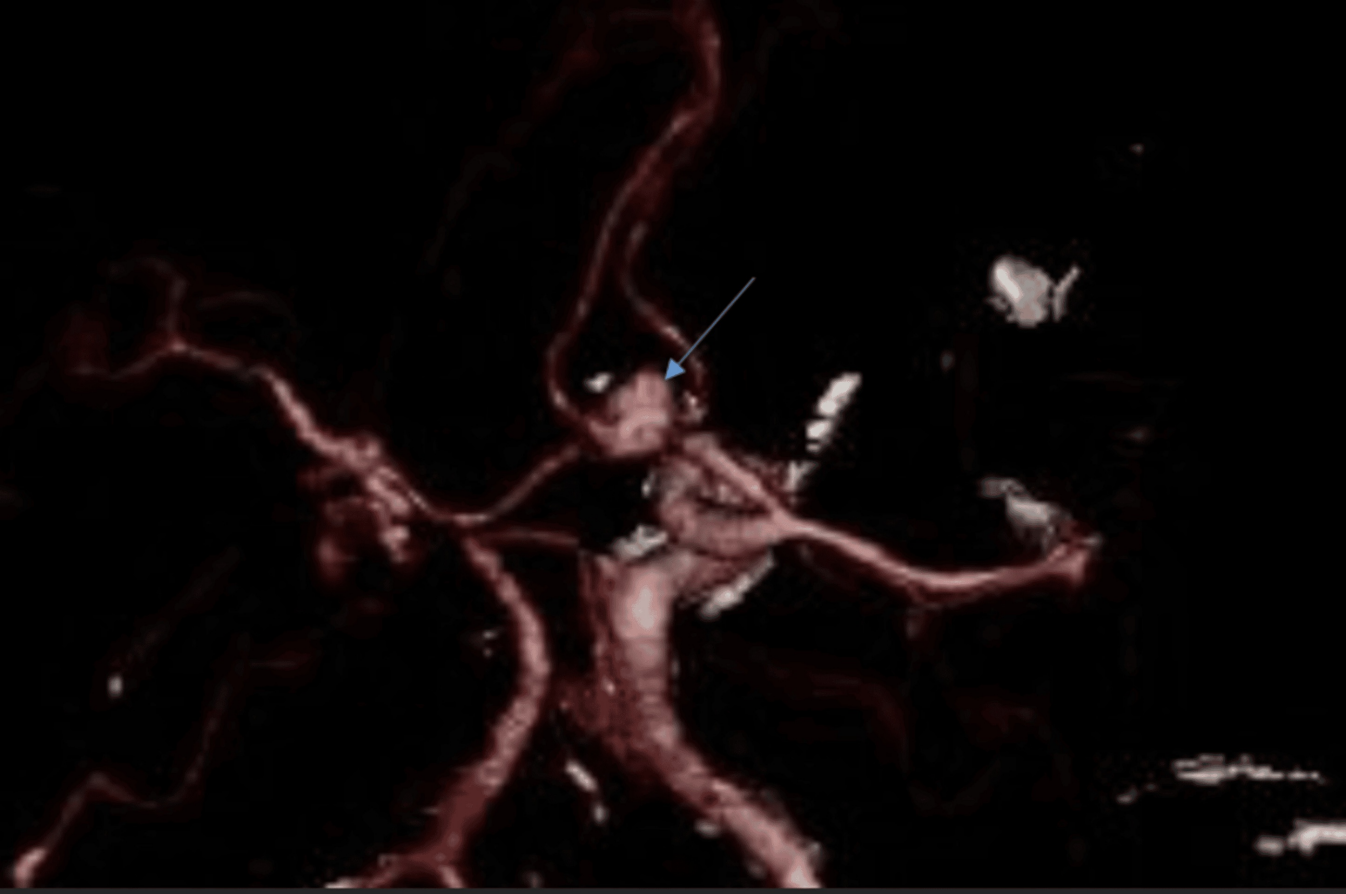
3D CTA showing the anterior communicating artery aneurysm.
